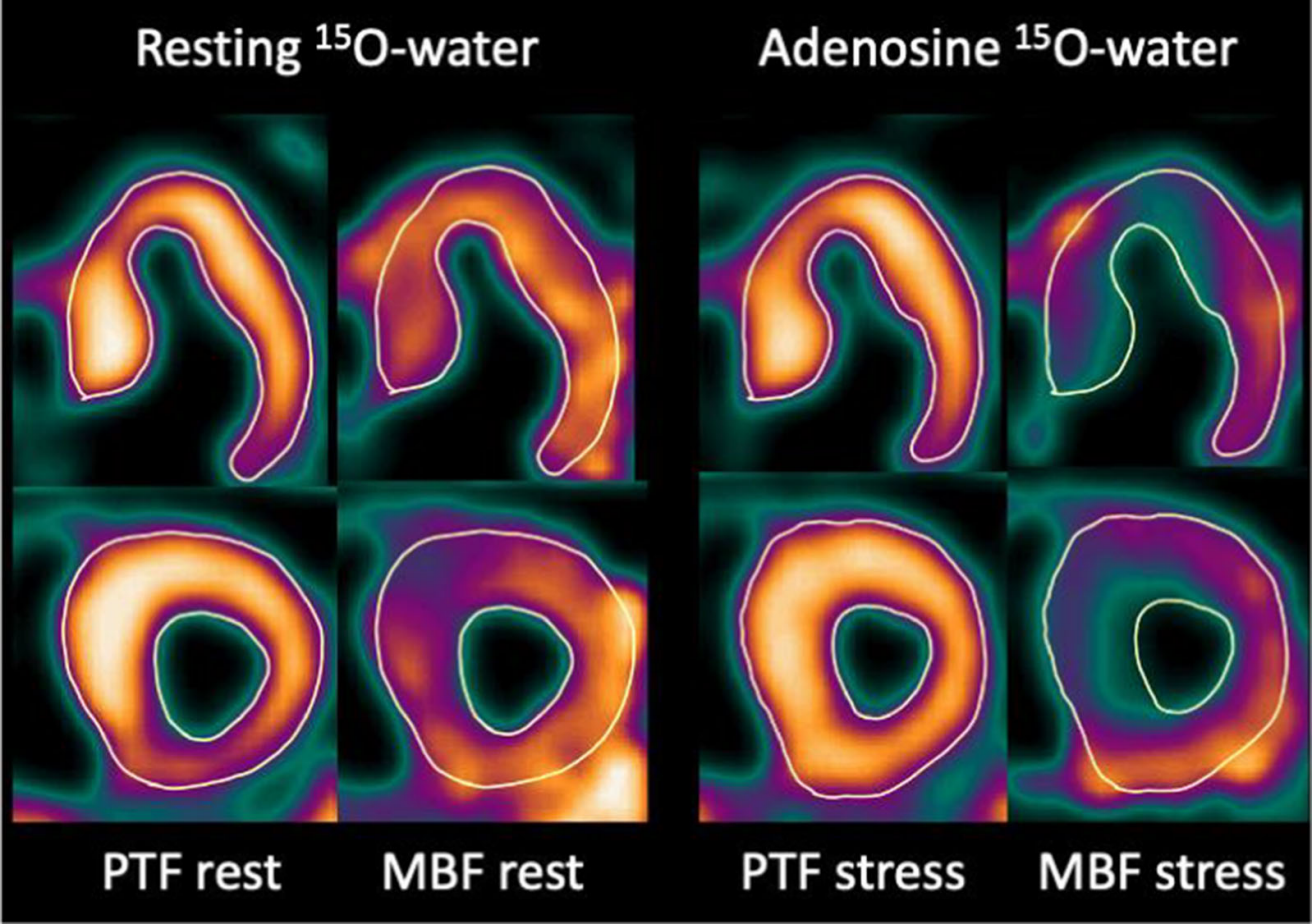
Example parametric ^15^O-water PET images of Perfusable Tissue Fraction (PTF) and myocardial blood flow (MBF) in a subject with hypertrophic obstructive cardiomyopathy, scanned at rest and during adenosine infusion stress. Rest MBF shows a mild perfusion defect in the anterior interventricular septum and stress MBF severe subendocardial perfusion deficits in septal and apical regions. PTF images at rest and stress are similar. Septal wall thickness by 27 mm PET and 25 mm by 2D-echocardiography
